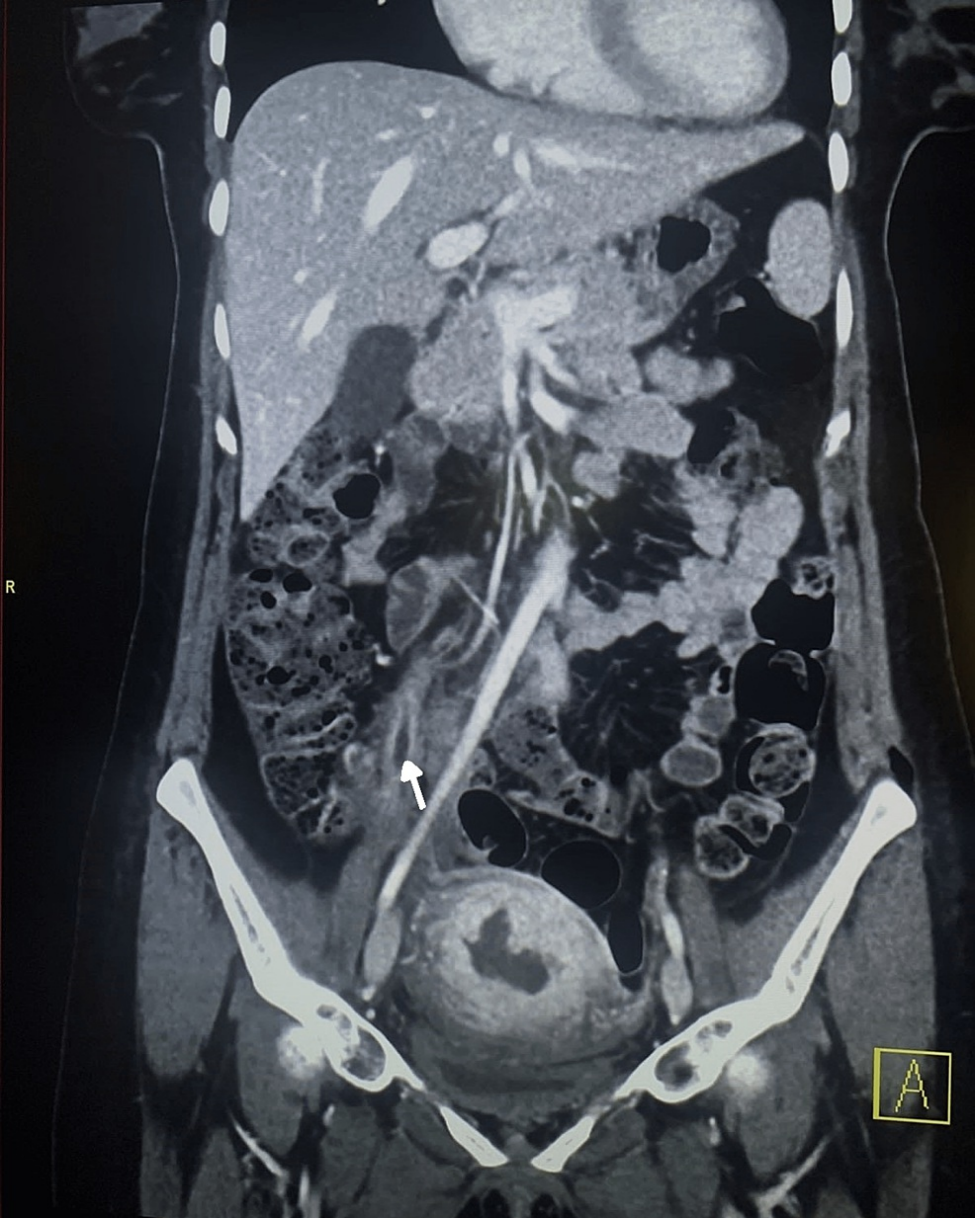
CT scan of the pelvis in Case 1 showing right ovarian vein thrombosis (arrow)
